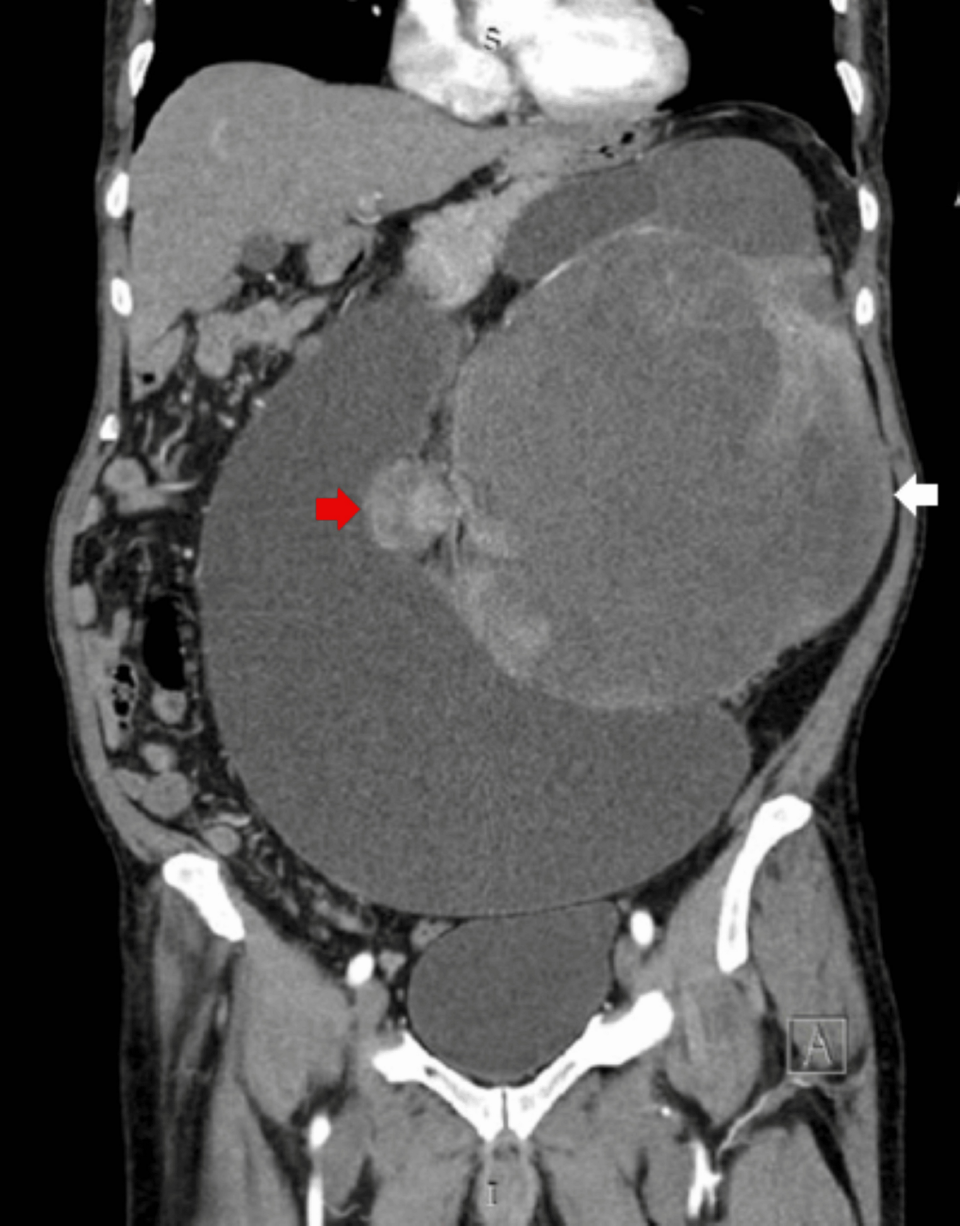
Contrast-enhanced CT (coronal view) of the abdomen demonstrating a large, heterogeneous mass replacing the left kidney. The mass demonstrates a peripherally enhancing soft tissue component with a large central area of necrosis (white arrow). Rounded, enhancing soft tissue lesion with central necrosis, measuring 4.5 × 4 cm, is seen anterior to the aorta below the origin of the renal arteries, exhibiting similar characteristics to the primary mass (red arrow)
